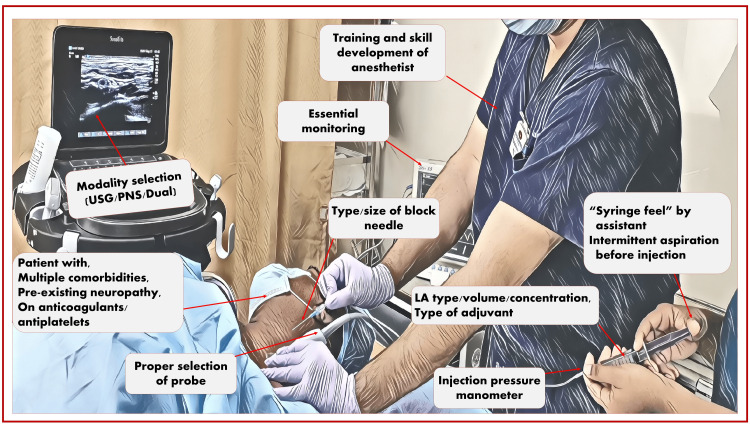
Important factors to prevent nerve injuries during the administration of peripheral nerve block. Source: This figure was created by the first author (K.S.). USG, ultrasound guidance; PNS, peripheral nerve stimulator; LA, local anesthetic
